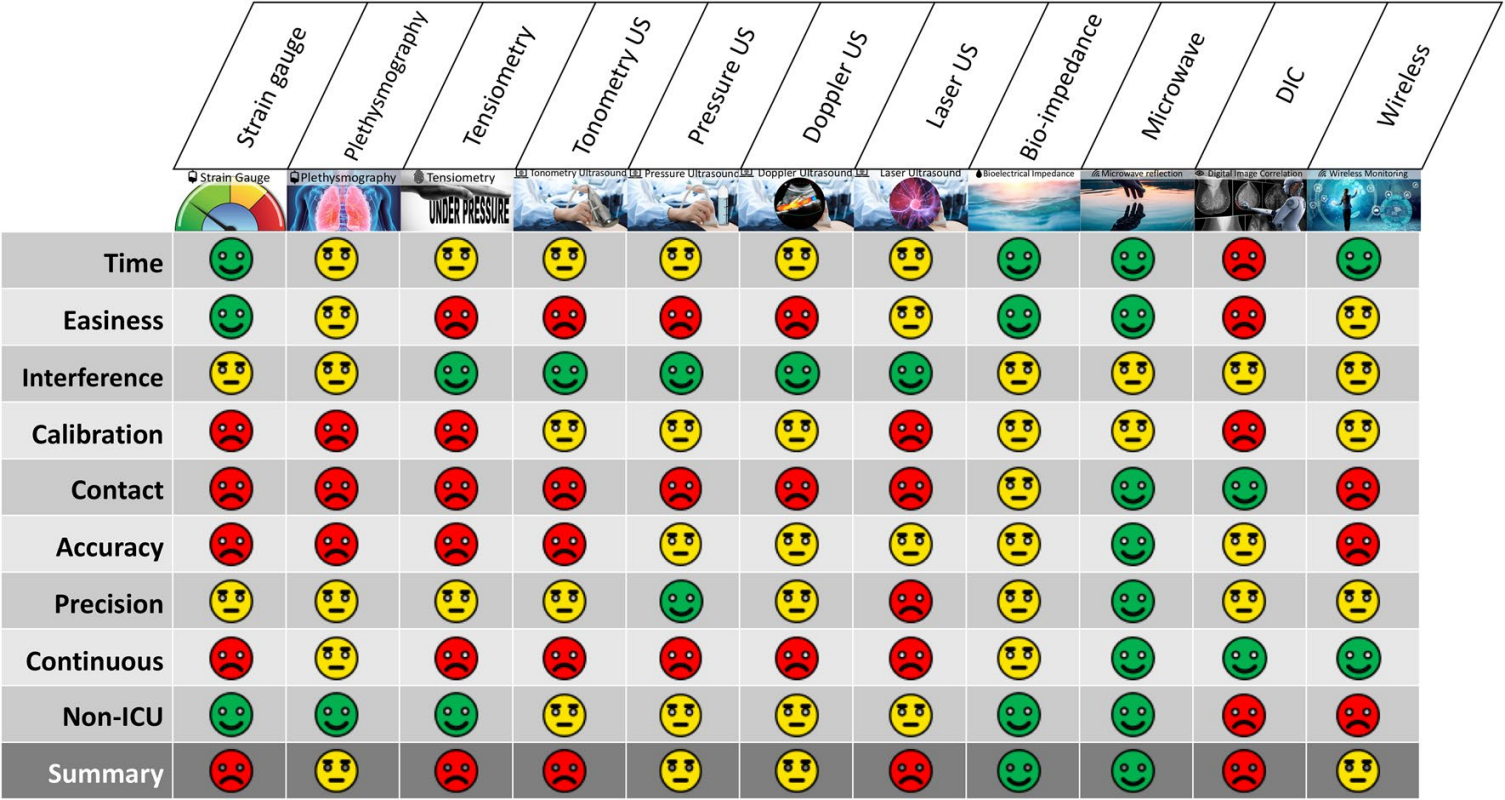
Summary of characteristics of the different non-invasive IAP measurement techniques. (DIC: digital image correlation, US: ultrasound)
